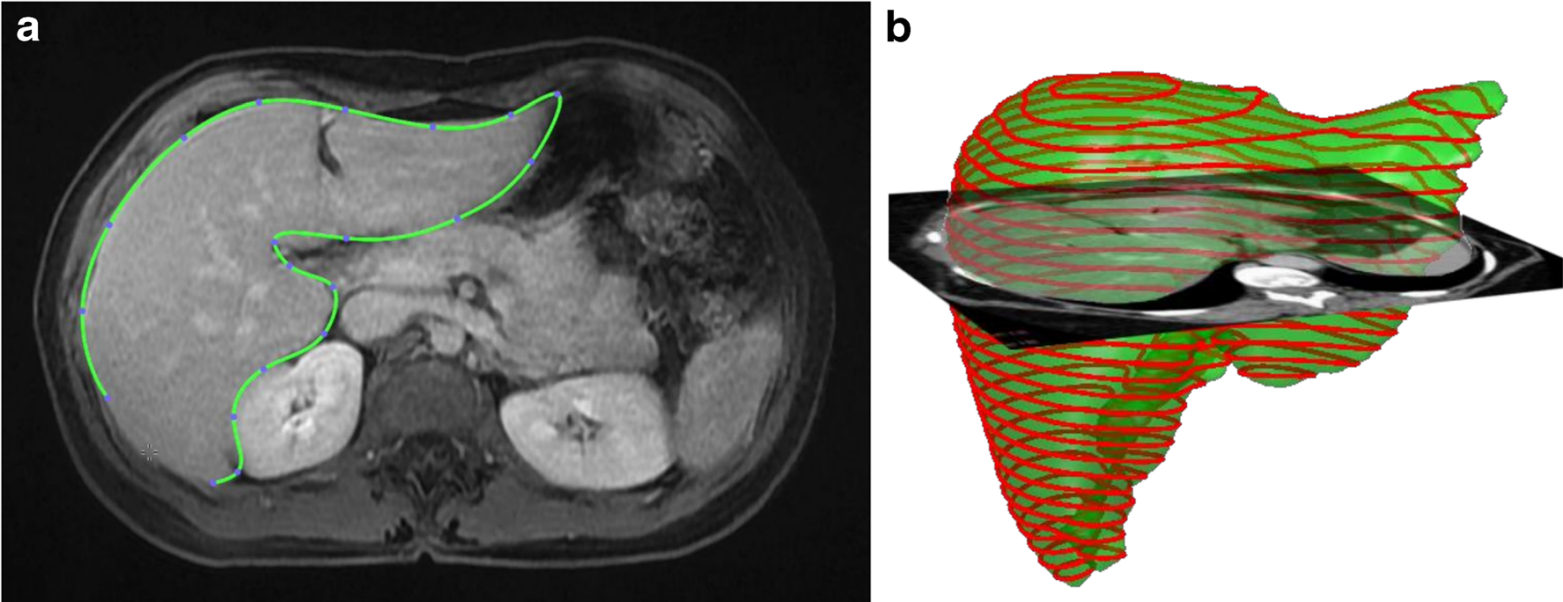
Manual segmentation of the liver. a Manual segmentation of the liver performed by contouring of pixels of the liver boundary on CT image. Image obtained using Osirix image post-processing software (Osirix Foundation, Geneva, Switzerland). b Volume of the liver is obtained based on pixel size and slice spacing [13]
